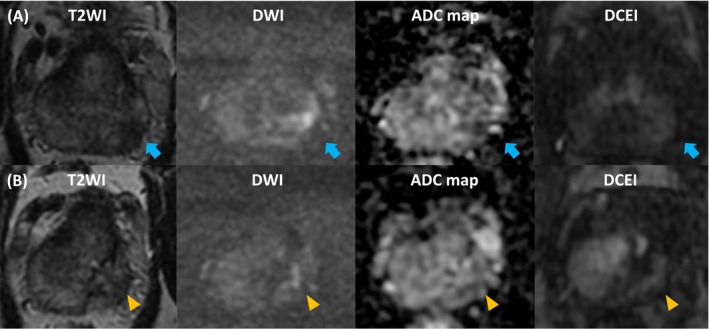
A Representative Case with High‐Visibility. A) A 55‐year‐old male with a PSA value of 5.3 ng/mL. Pre‐biopsy multiparametric MRI identified a 12 mm PIRADS score four lesion (blue arrowhead) at the left base peripheral zone of the prostate. Targeted biopsy toward the lesion confirmed GG 2 prostate cancer with cancer involvement of 90%. The patient underwent left hemigland HIFU. B) Follow‐up MRI at 1 year revealed a small area of focal nodular enhancement (orange triangle) in the region of the previous treatment. GG 2 prostate cancer recurrence was detected on targeted follow‐up biopsy of the lesion. The patient underwent salvage left hemigland HIFU, followed by no GG ≥ 2 recurrence over 2 years. Repeat PGA is a preferred option for unifocal GG 2–3 recurrence with limited cancer volume, long life expectancy, and the patient's desire to preserve urogenital functions.^16^ GG, Gleason grade group; HIFU, high‐intensity focused ultrasound; MRI, magnetic resonance imaging; PGA, partial gland ablation; PIRADS, Prostate Imaging Reporting & Data System; PSA, prostate‐specific antigen.
